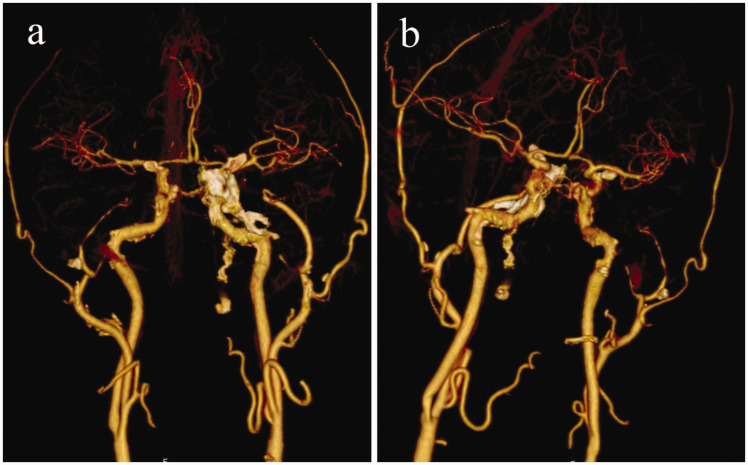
Computed tomography angiography images. (a, b) Images showing mixed plaque formation in the V4 segment of the right vertebral artery, combined with mild stenosis of the vessel lumen; soft plaque formation in the V2–V4 segments of the left vertebral artery, combined with occlusion of the vessel lumen; soft plaque formation in the middle and distal segments of the basilar artery, combined with occlusion of the vessel lumen; and mixed plaque formation in the P1 segment of the left posterior and right superior cerebellar arteries, with severe stenosis of the vessel lumen.
